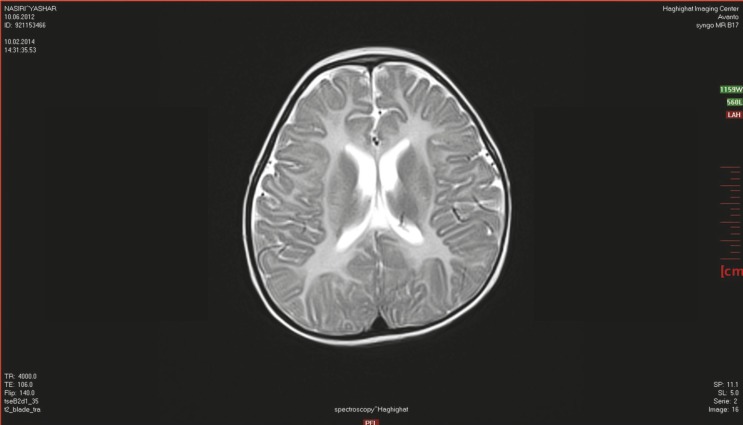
Canavan’s disease demonstrates bilateral symmetric T2 white matter hyperintensity including involvement of the subcortical arcuate fibers. This disease appears diffusely throughout the cerebral white matter and does not enhance in computed tomography (CT) or MR imaging, and demonstrates variable involvement of the basal ganglia and cerebellar white matter
